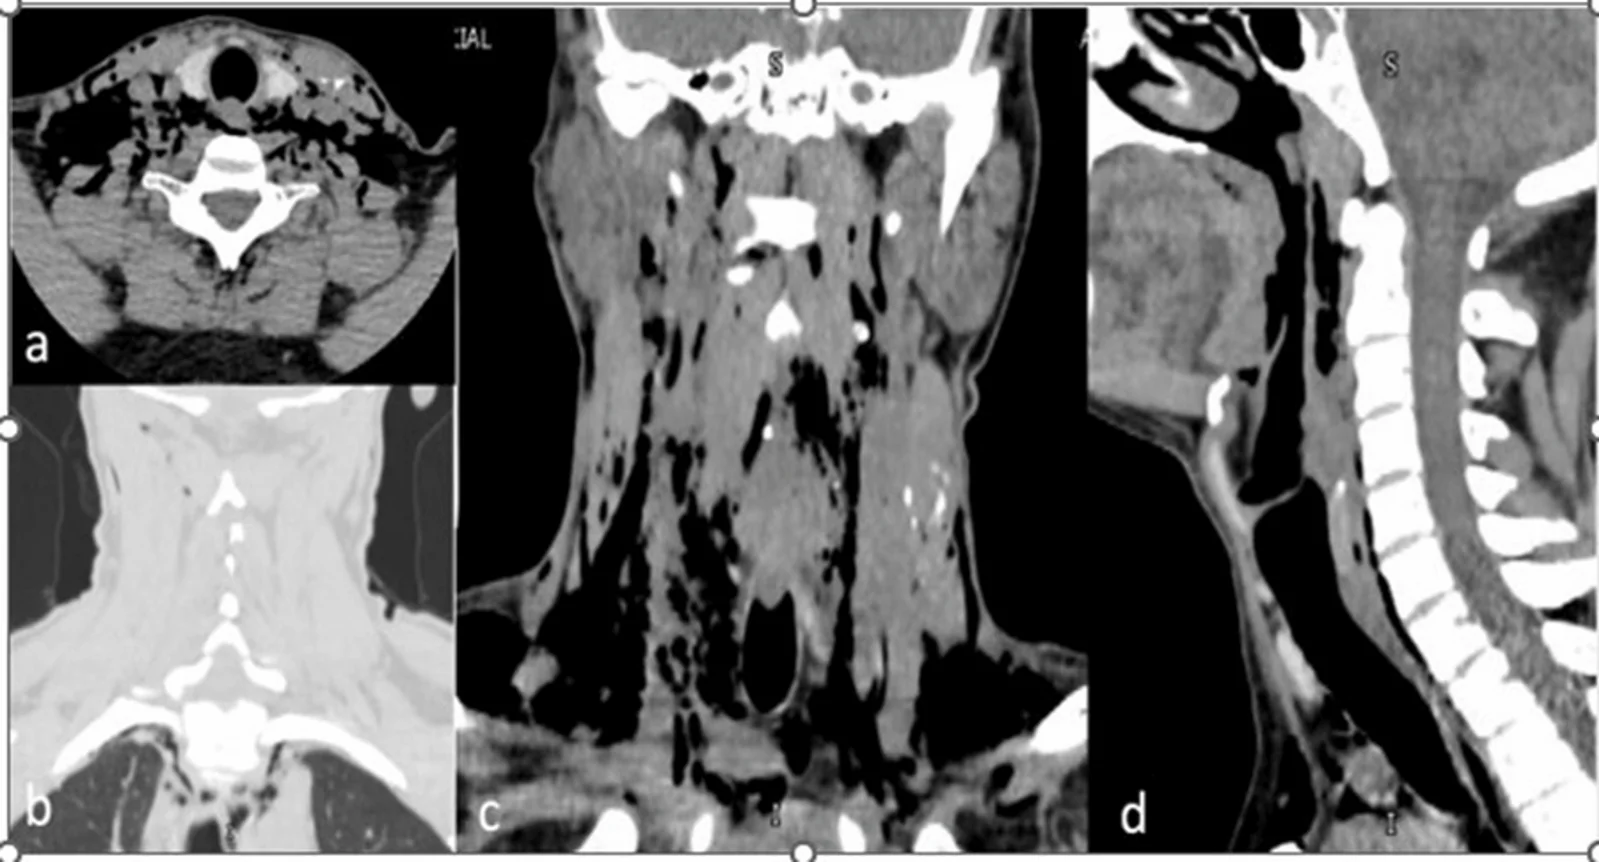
Axial (a) and coronal (c) views of computed tomography showing extensive surgical emphysema with air dissecting soft tissue planes of the neck. (b) Coronal view showing pneumomediastinum. (d) Sagittal view showing intact airways
